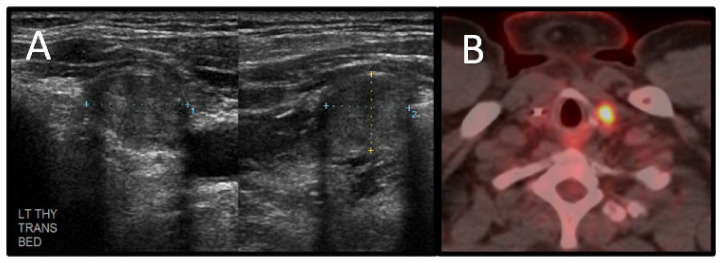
A 43-year-old man with a history of Cowden syndrome and follicular thyroid cancer diagnosed at age 30. The patient underwent thyroidectomy at diagnosis. He subsequently was diagnosed with primary lung cancer and was found to have a nodule in the resection bed on routine surveillance. (A) Grayscale ultrasound demonstrates a well-circumscribed nodule involving the left thyroid resection bed suggestive of recurrence. (B) Nodule shows increased FDG uptake on positron emission tomography/computed tomography. The nodule was biopsied and found to represent follicular thyroid carcinoma.
